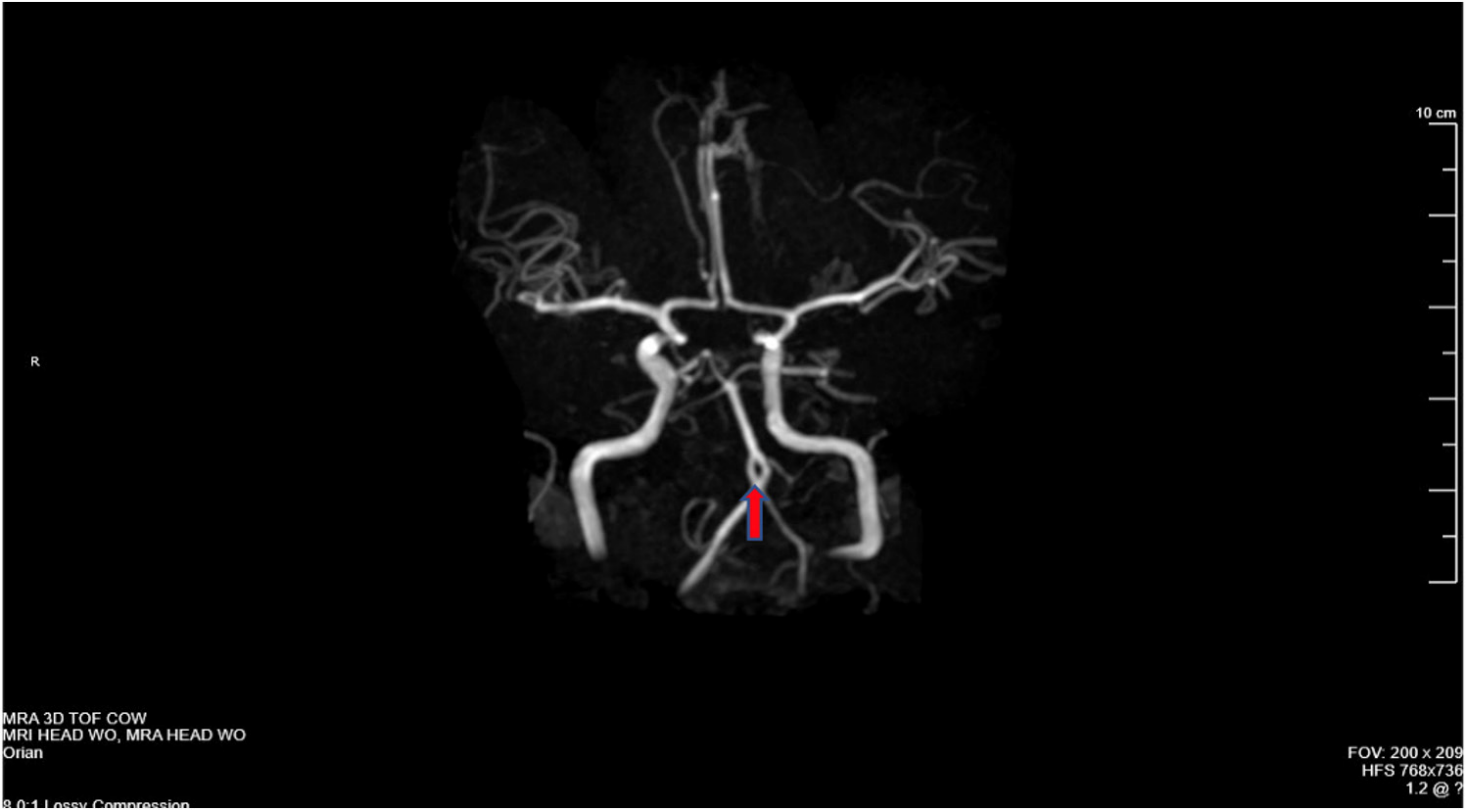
Magnetic resonance angiography (MRA) Head without contrast highlights basilar artery fenestration measuring 6 mm in craniocaudal diameter. The red arrow is pointing to the anatomic finding. No other intracranial abnormalities were noted. Magnetic resonance imaging/angiography (MRI/MRA) revealed the characteristic features of basilar artery fenestration, with two parallel channels of the basilar artery separated by a septum, rejoining distally to form a single basilar artery. No evidence of thrombosis, aneurysm, or other associated vascular abnormalities was noted.
